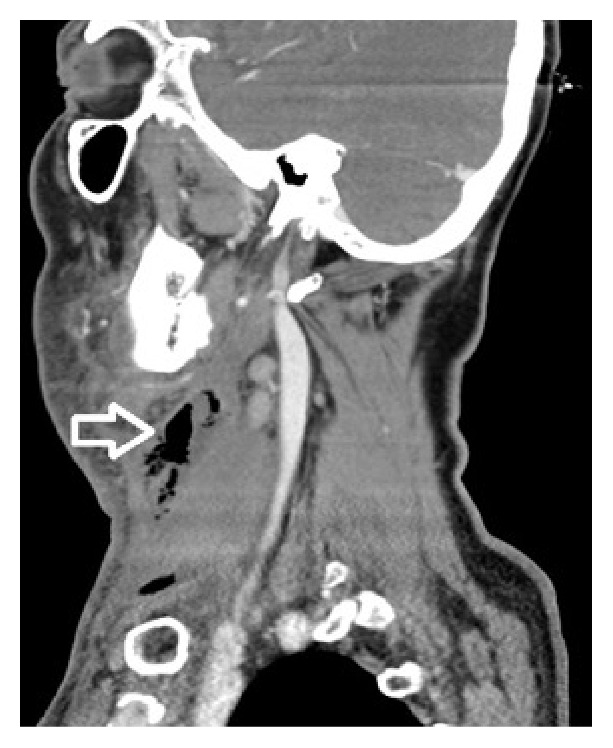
Sagittal neck CT image showing cervical subcutaneous gas (white arrow).
